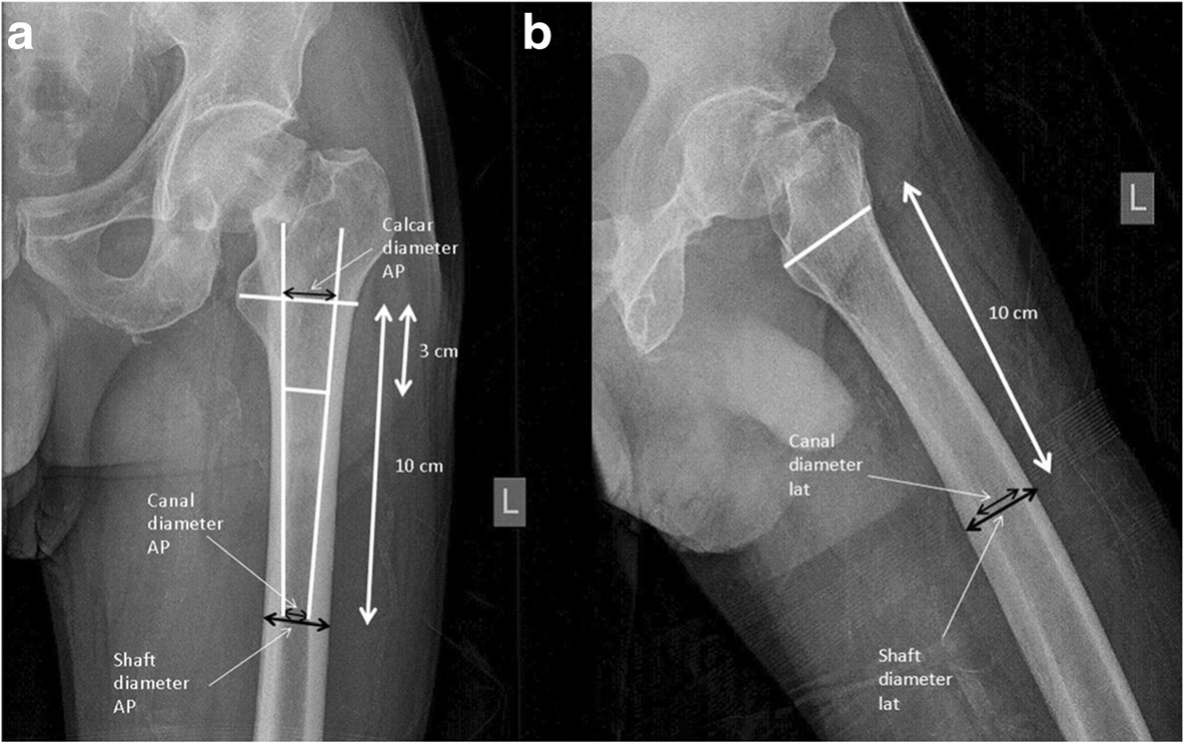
Antero-posterior view (a) and lateral (b) radiographs of the hip demonstrating the area of radiographic measurements
